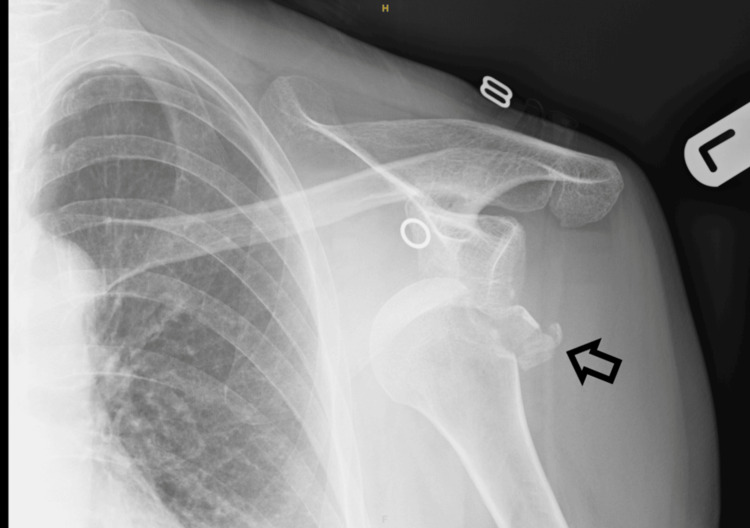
X-ray of the left shoulder in AP view demonstrates a fracture-dislocation of the proximal humerus
